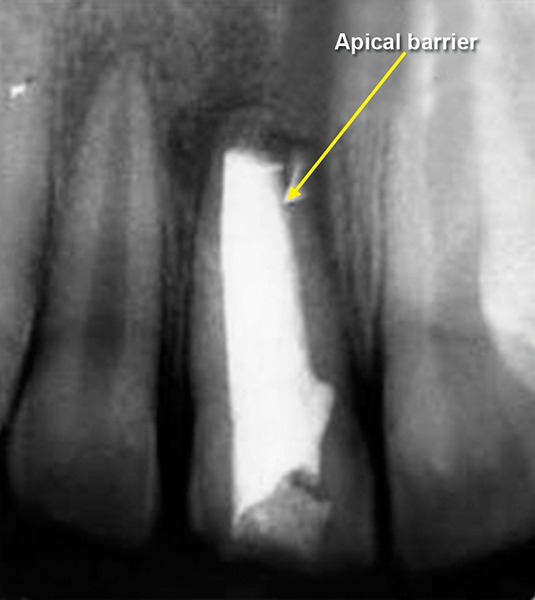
Intraoral periapical radiograph after 6 months showing apex fully sealed
